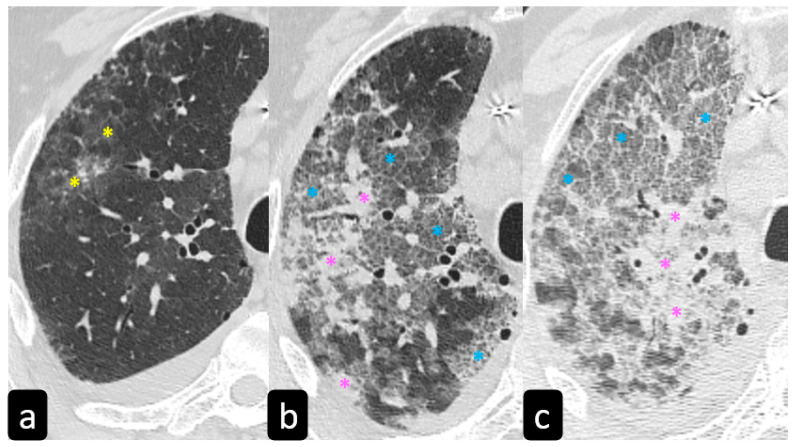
Patient presenting with fever and dyspnea. (a) At the first chest CT scan, day 2 showed patchy ground-glass opacities (yellow *). (b) At day 8, the lesions evolved intoconsolidations (pink *) and “crazy-paving” pattern lesions (blue *) and at peak time, day 14, became larger (c).
